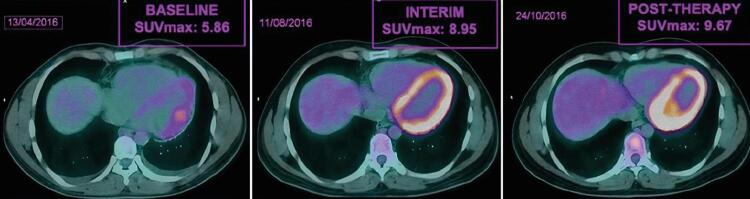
Exemplo de caso - valor máximo de captação (SUV, do inglês standardized uptake value) (SUVmax) no ventrículo esquerdo na tomografia computadorizada por emissão de pósitron (PET/CT) basal (5,86), intermediário (8,95/52,73% de aumento a partir do basal) e pós-terapia (9,67/65,02% de aumento a partir do basal).
